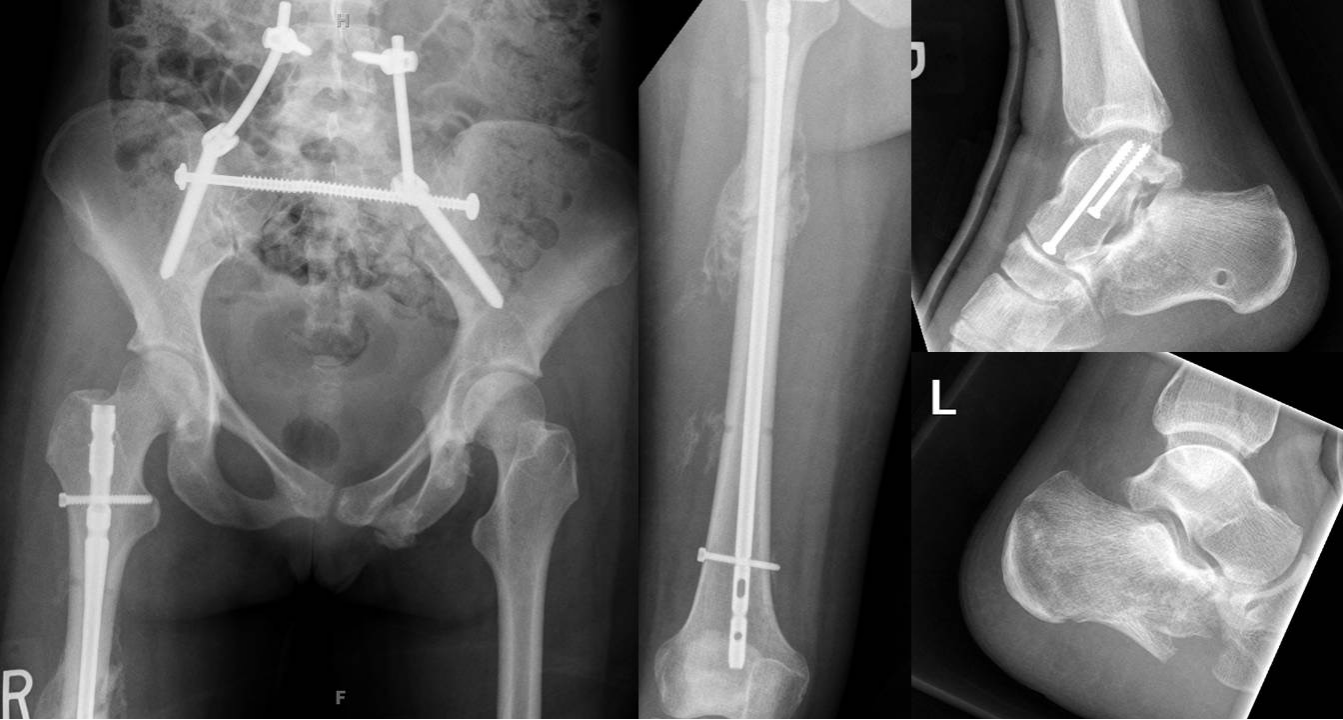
Early fracture healing documented by X-rays obtained at 6 weeks post injury.
